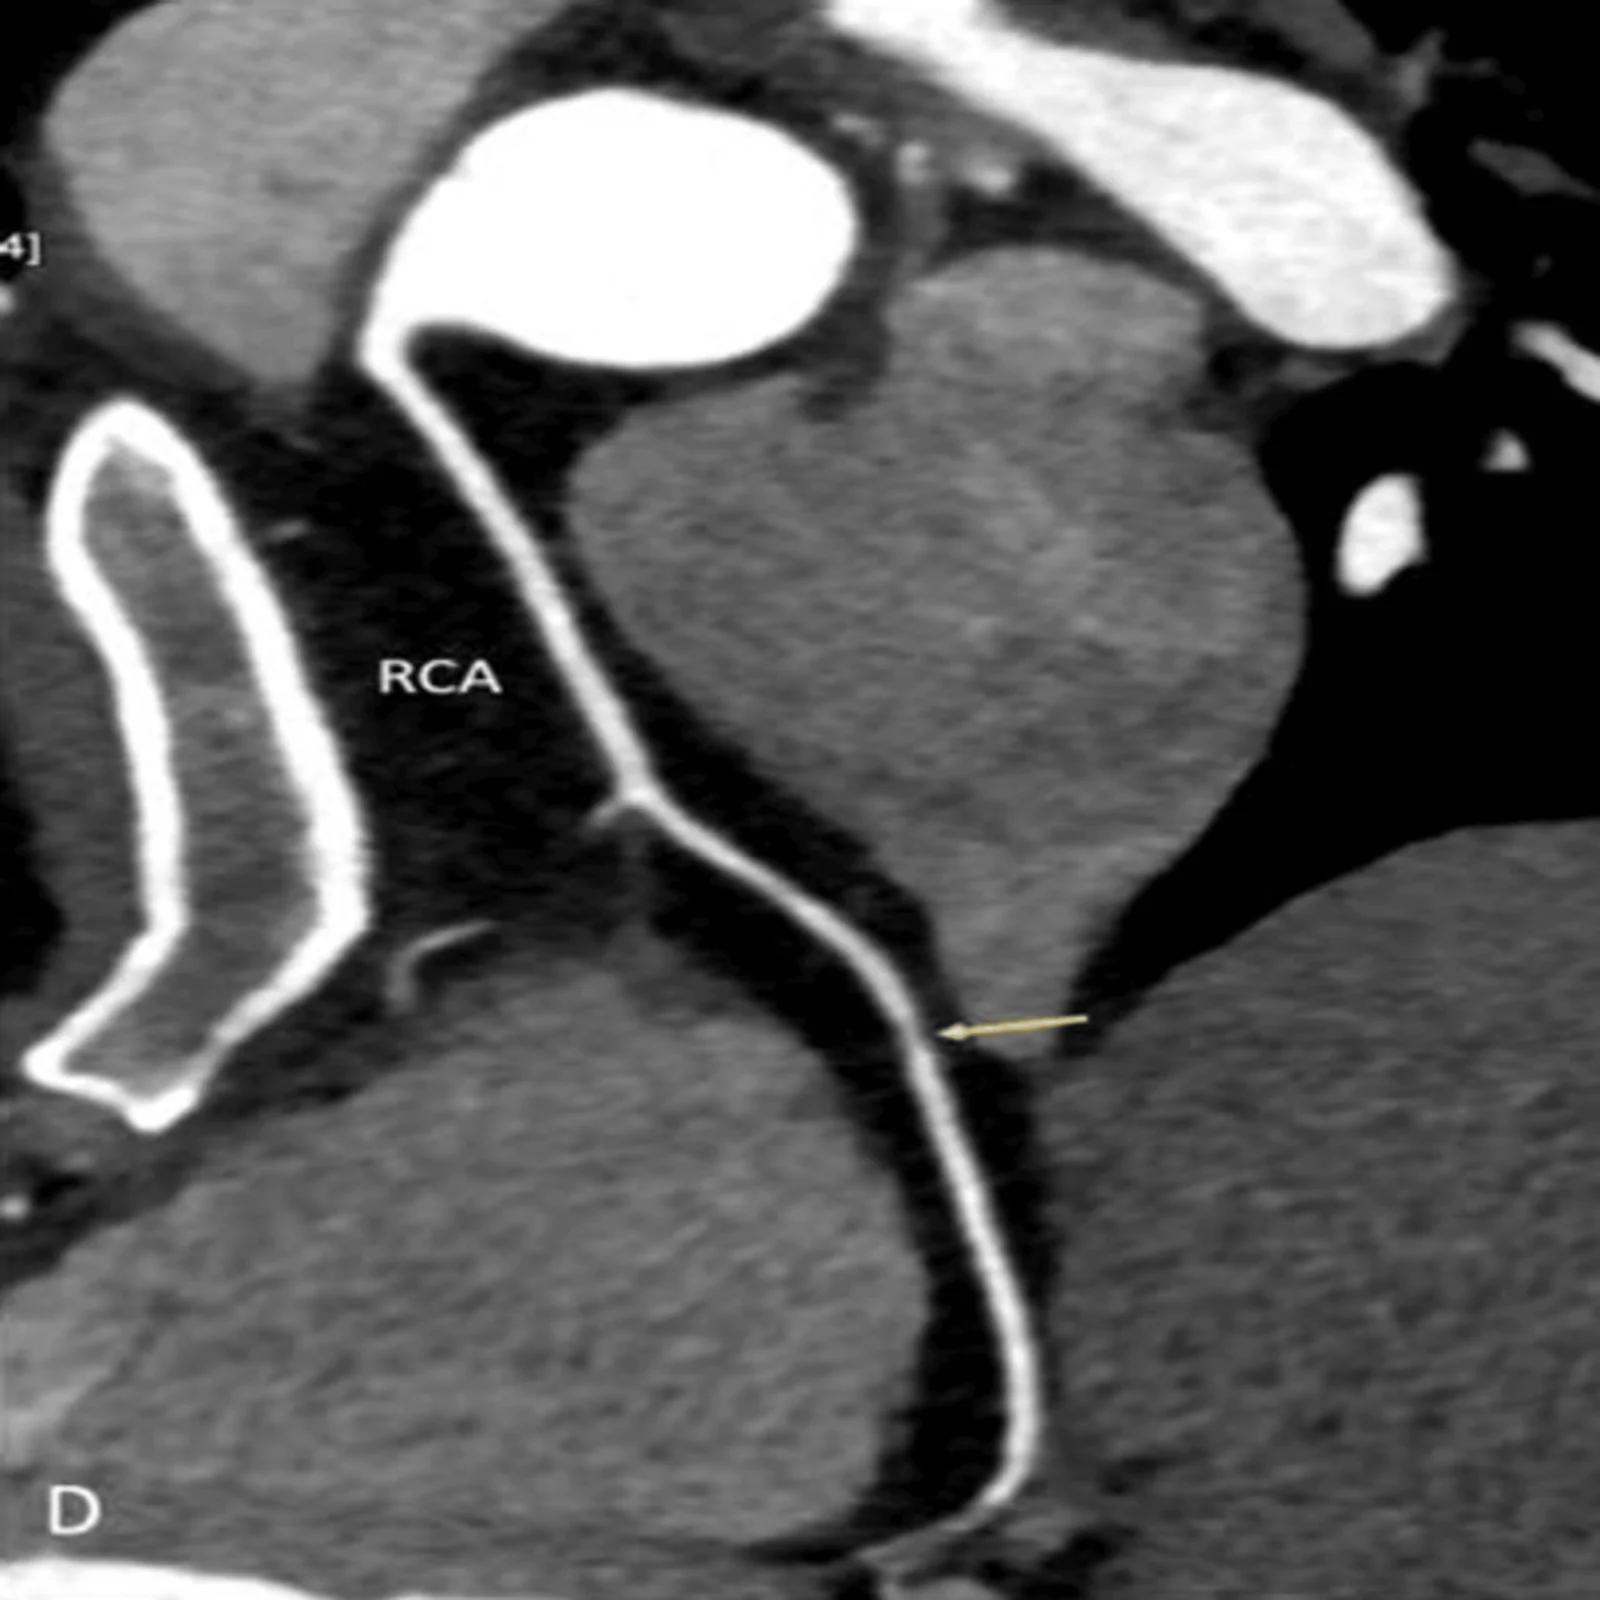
CT coronary angiography (curved planar reformation; Syngo.via) of the right coronary artery (RCA). The arrow marks a significant stenotic segment contributing to elevated Synergy Between Percutaneous Coronary Intervention With Taxus and Cardiac Surgery (SYNTAX) and Gensini scores in this patient. Syngo.via workstation (Siemens Healthcare GmbH, Erlangen, Bavaria, Germany).
